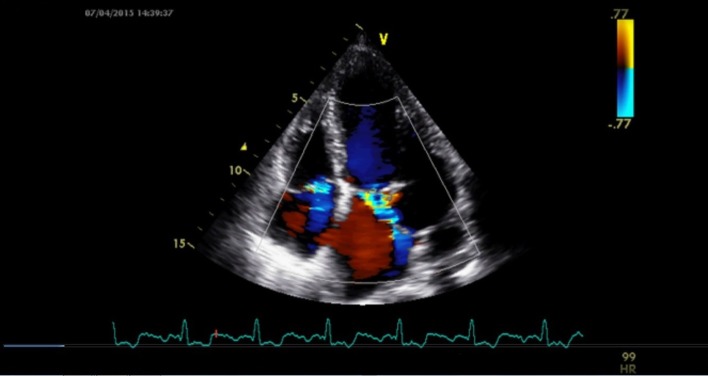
Color Doppler showed severe MR with an eccentric jet with severe tricuspid regurgitation.
